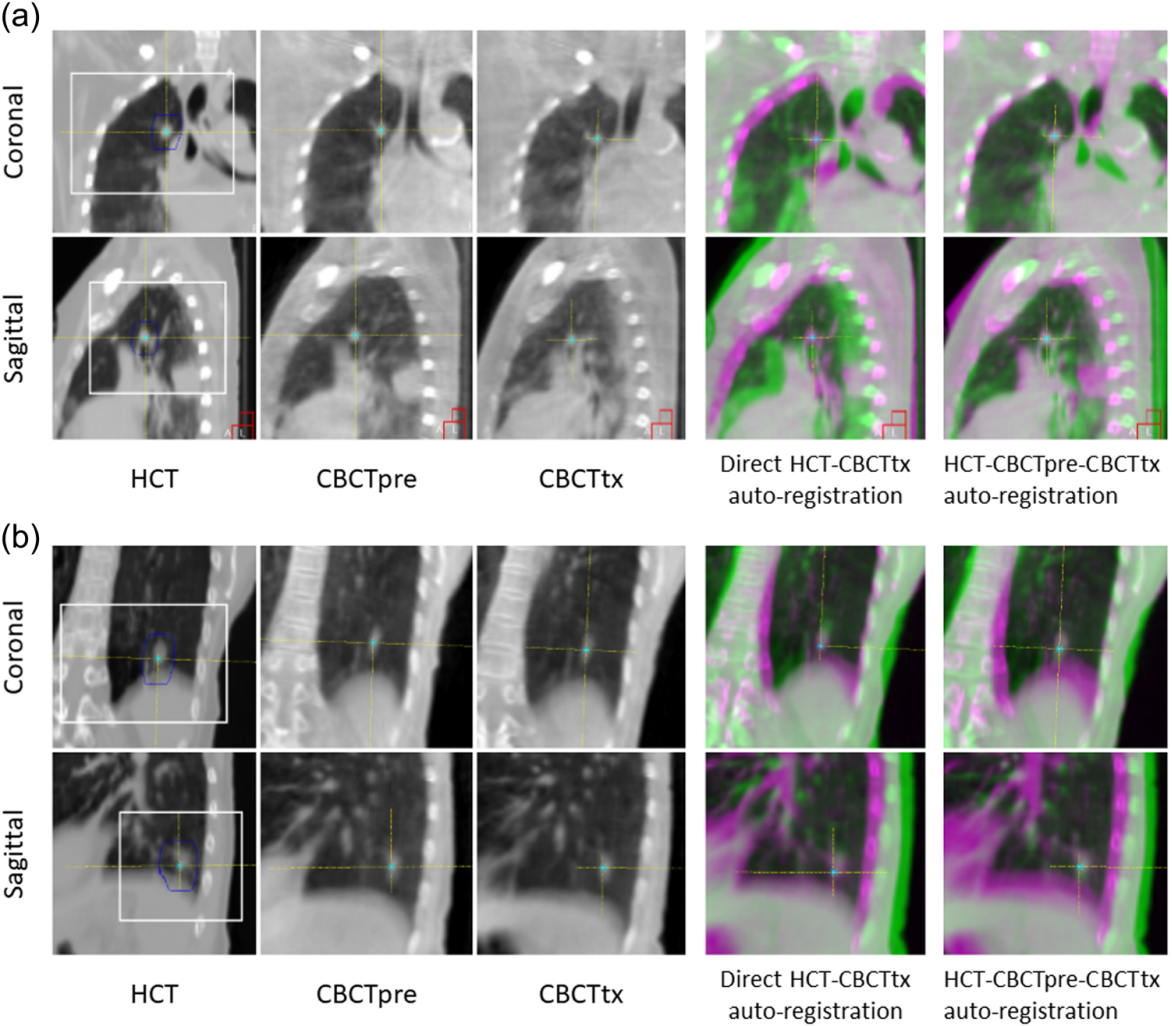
Images of the reference helical CT (HCT), cone beam computerized tomography (CBCT)pre, and CBCTtx for patient #29 (a) and patient #32 (b). The clip box (white) and mask (blue) regions of interest used during dual registration are displayed on the HCT images. The color blended images show the registration results between reference HCT (purple color) and CBCT (green color) from two auto‐registration methods
